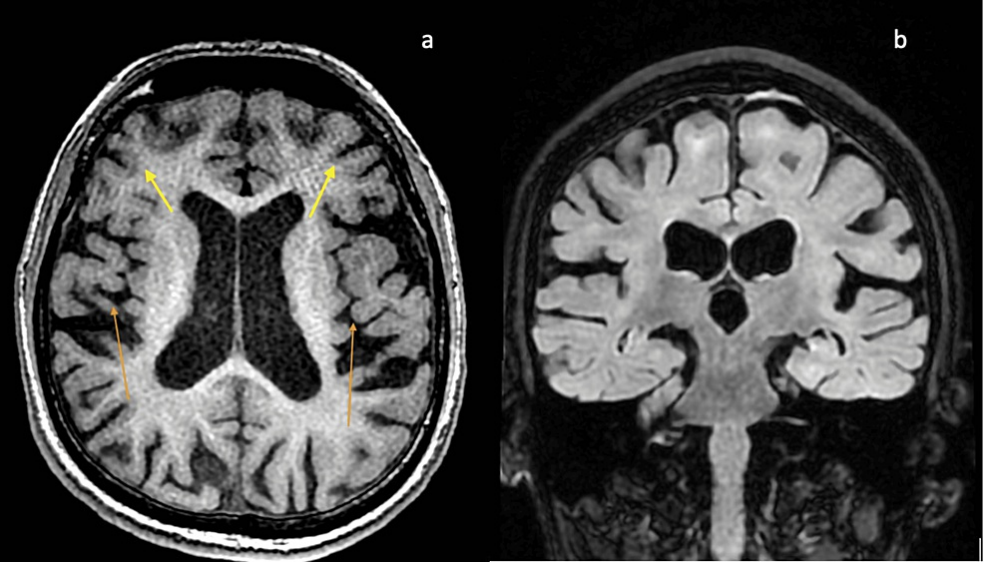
MRI of the brain images of the patient (3a) T1 axial weight image of the MRI of the brain showing diffuse cerebral atrophy disproportionate for age, predominantly in the bilateral temporal (orange arrows) and the frontal regions (yellow arrows). (3b) T1 coronal MRI of the brain demonstrating bilateral frontal and temporal atrophy disproportionate for age.
